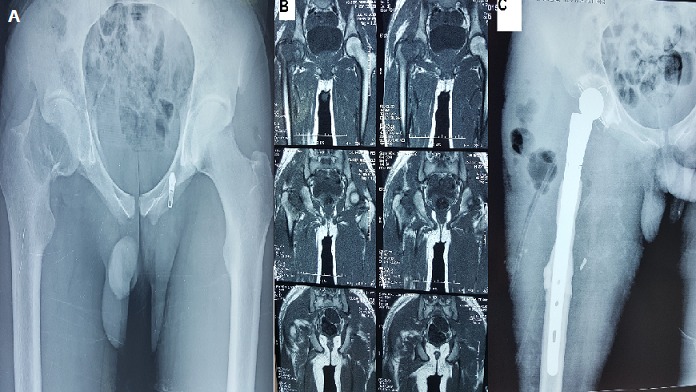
(A) radiographie du bassin objectivant une fracture du col fémoral droit sur os pathologique; (B) scanner du bassin montrant une fracture du col fémoral droit avec une ostéolyse du col et de la tête fémorale; (C) radiographie de contrôle de la hanche droite après la résection tumorale et la reconstruction par une prothèse massive
